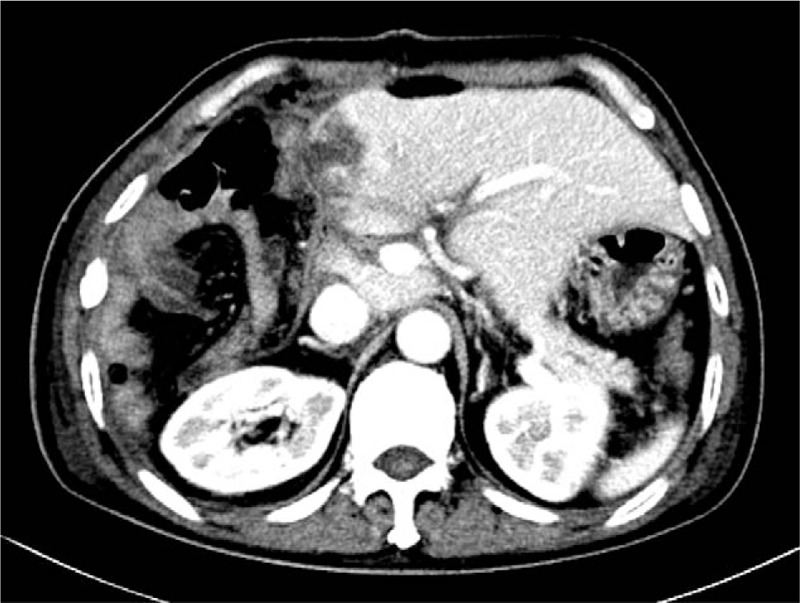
Computed tomography: the liver after right hemihepatectomy. Postoperative recovery was uneventful.
